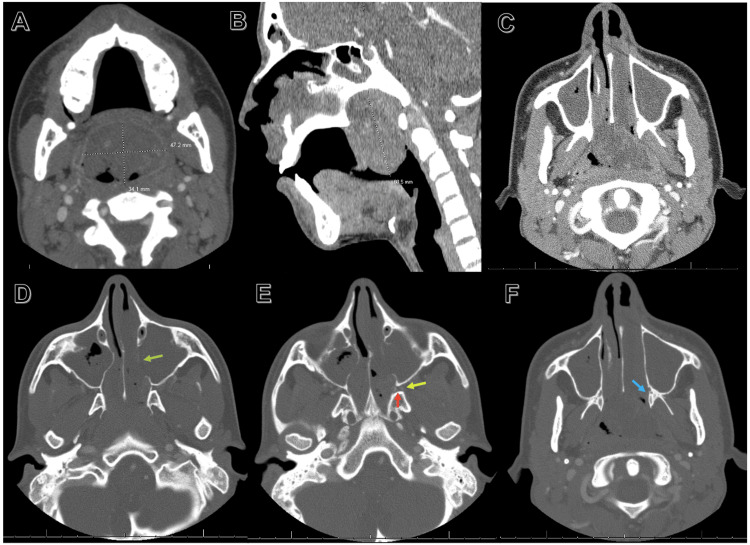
CT maxillofacial/mandible with contrast (A-C) A large mass centered at the nasopharynx measuring 3.4 x 4.7 x 6.1 cm. Internal serpiginous foci of hyperdensity were noted within the mass resembling hypervascularity. The mass extends anteriorly through the nasal choanae and inferiorly to the level of the tongue, displacing the uvula anteriorly. There is a resultant narrowing of the airway at this level. (D) Mixed attenuation complex fluid filling the maxillary sinuses. The left maxillary sinus wall appears eroded (green arrow). (E) Pterygopalatine fossa (red arrow) and pterygomaxillary fissure (yellow arrow), which are unchanged in size when compared with the right side. (F) Medial pterygoid plate (blue arrow), which is unchanged when compared with the right side.
